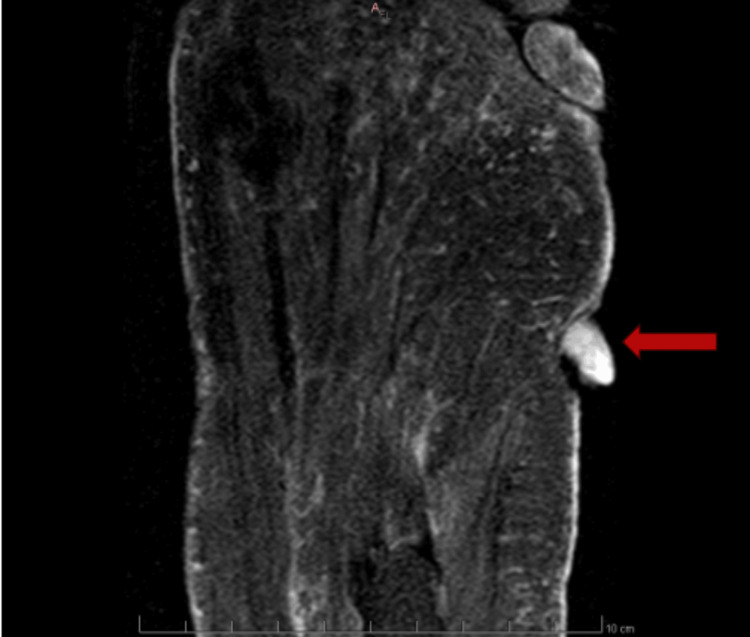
Axial STIR MRI of the left foot demonstrating a hyperintense pedunculated mass (arrow) overlying the lateral aspect of the foot STIR: short tau inversion recovery; MRI: magnetic resonance imaging
